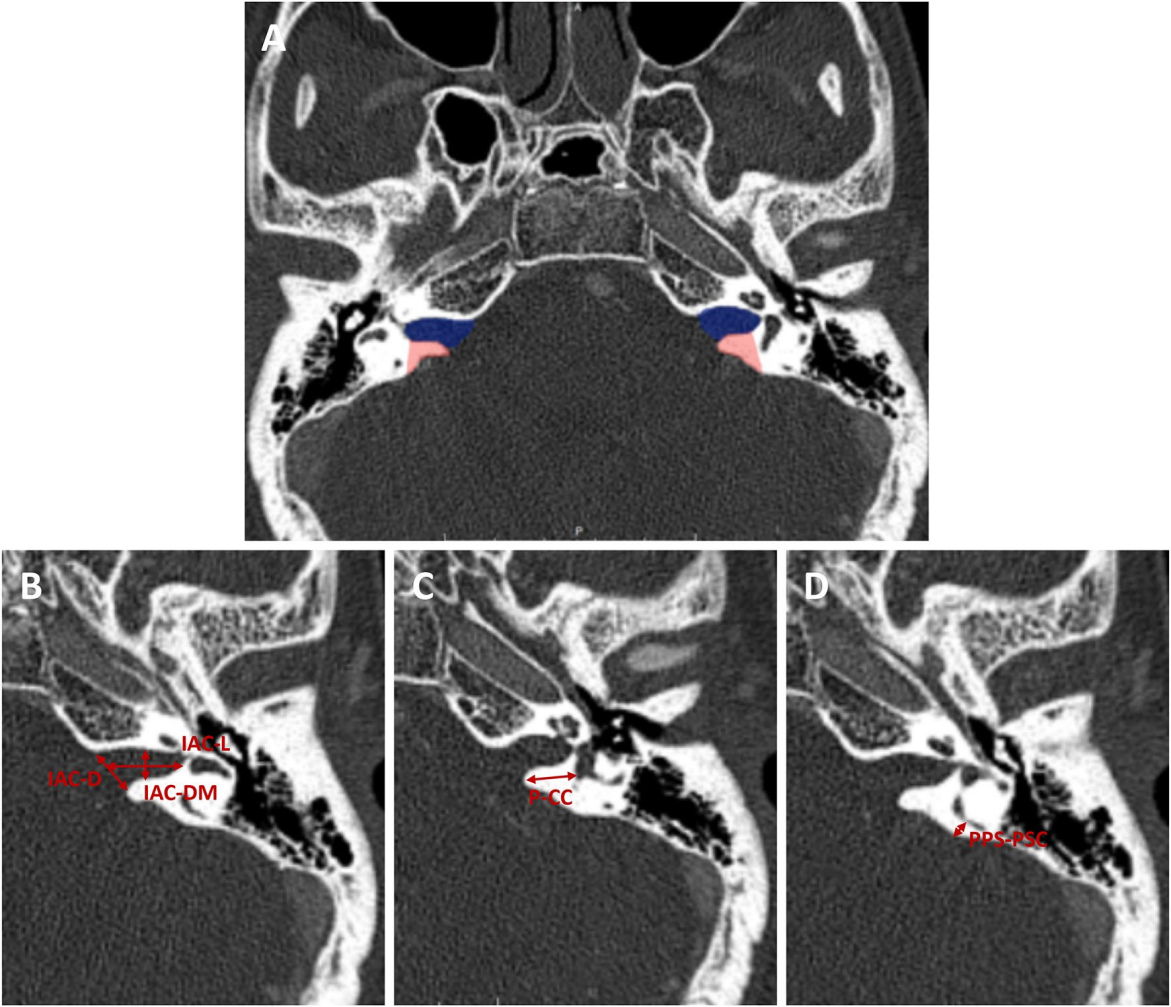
IAC and posterior petrous bone parameter measurements and surgical relevance. (A) Measurements of the IAC (highlighted in blue) and surgically relevant aspects of the posterior petrous bone were made from high-resolution CT scans. The area in red highlights bone removed during a retrosigmoid transmeatal approach, defined largely by the depth of the labyrinth from the posterior lip of the porous and the posterior petrosal surface (P-CC and PPS-PSC). (B) IAC length (IAC-L) was measured from the IAC porus to the fundus, along the center of the long axis of the IAC. IAC porus diameter (IAC-D) was measured from the anterior lip to the posterior lip of the IAC porus. IAC mid-point diameter (IAC-DM) was measured from the anterior to the posterior IAC at the midpoint between the porus and fundus (defined as the midpoint of IAC-L). (C) The distance from the porus to the common crus (P-CC) was measured from the posterior aspect of the IAC porus to the CC. (D) The distance from the posterior petrosal surface to the posterior semicircular canal (PPS-PSC) was measured from the posterior petrous face to the mid-aspect of the posterior semicircular canal.
